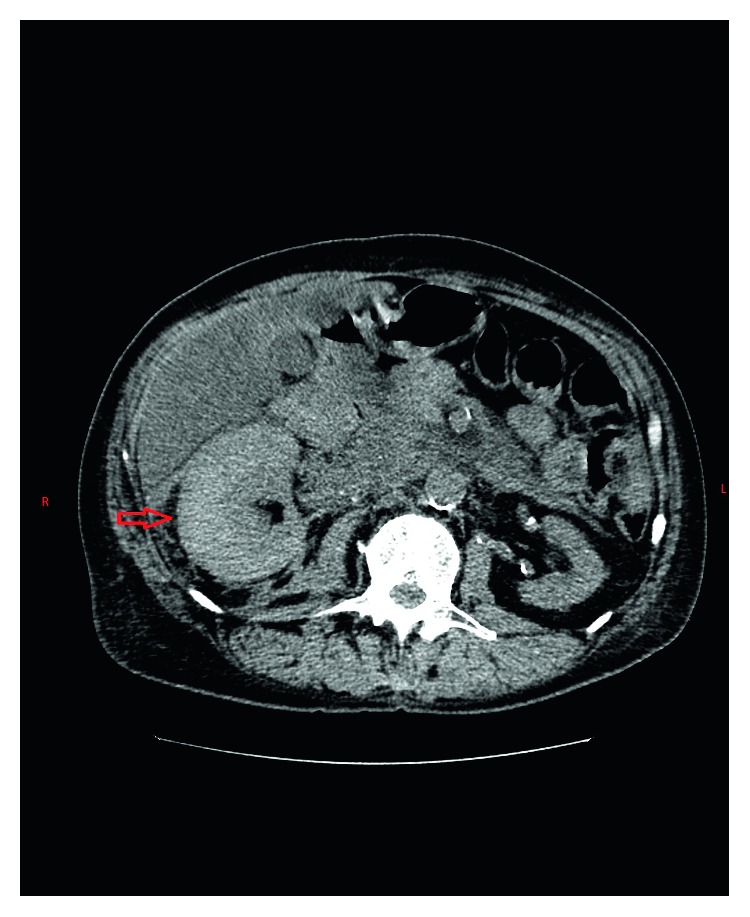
Axial section of noncontrast CT scan of the abdomen showing an enlarged right kidney (marked in red arrow) suggesting a potential collection within or outside the renal capsule.
